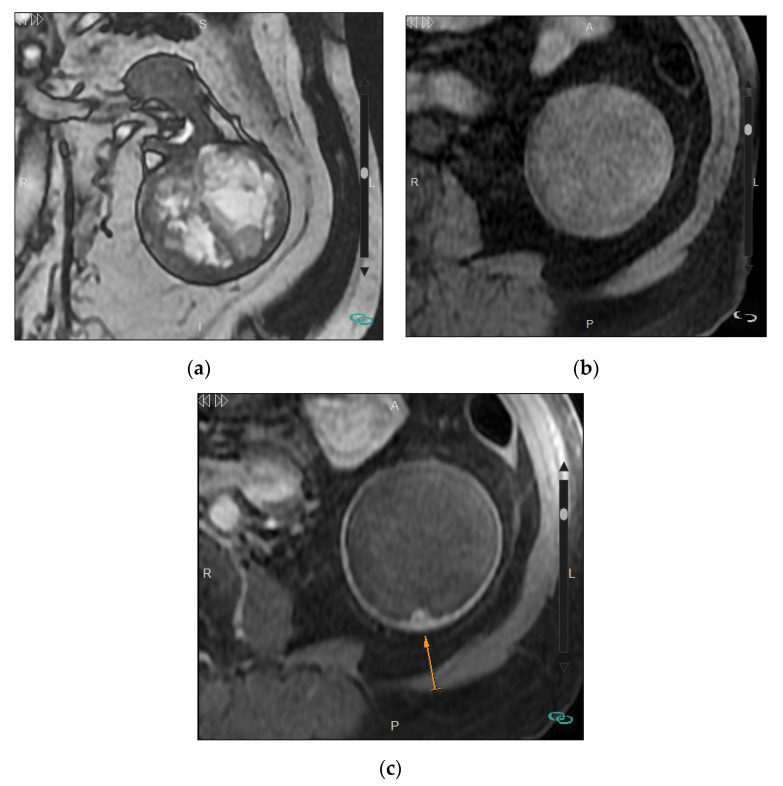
Bosniak IV cyst in a 77-year-old man. (a) Coronal fast spin-echo T2-weighted MRI shows a heterogeneous well-defined mass at the lower pole of the left kidney. (b) Pre-contrast T1-weighted image shows minimal heterogeneity of the mass. (c) Post-contrast T1-weighted image shows an 8 mm enhancing nodularity (arrow) arising from the posterior wall of a cystic mass corresponding to a Bosniak IV cyst.
